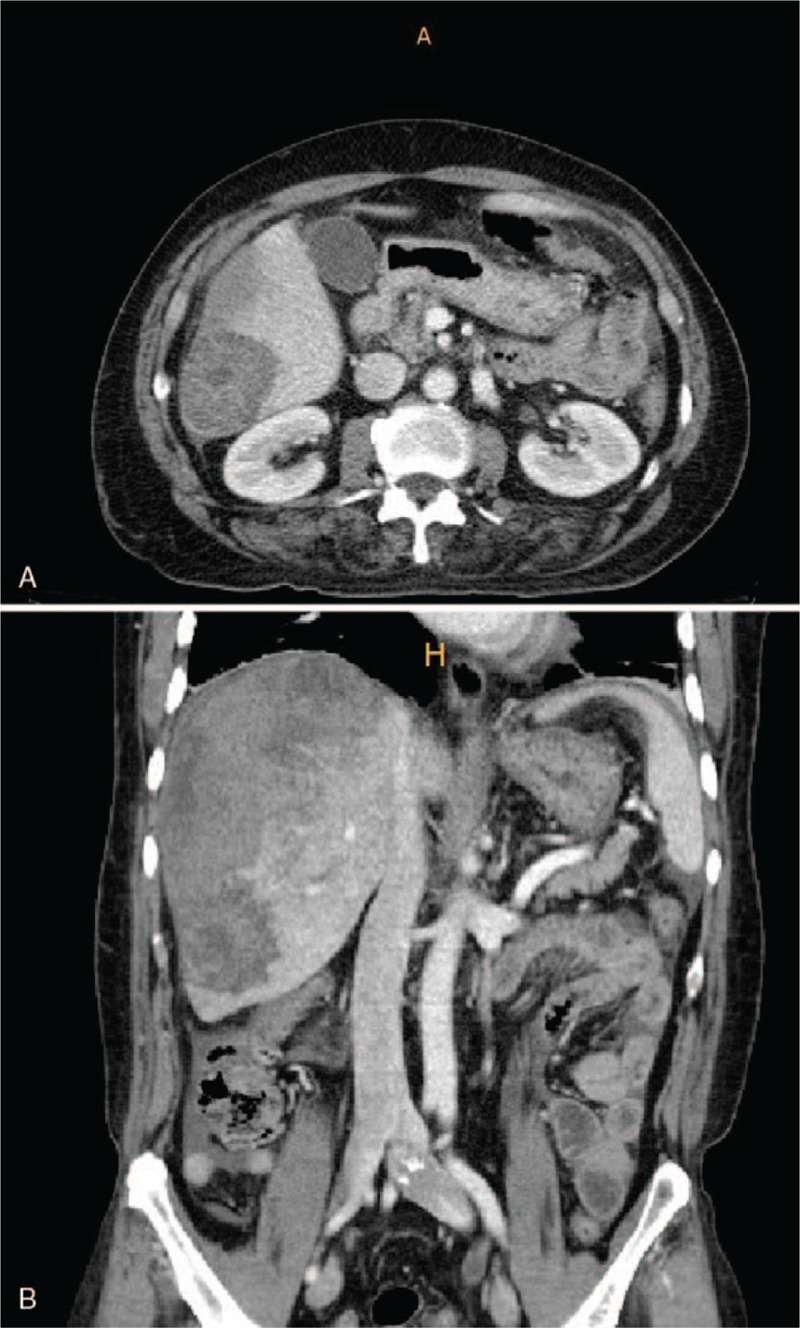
Computed tomography showed a hypodense mass in segment VI of the right hepatic lobe. There is no pneumoperitoneum.
